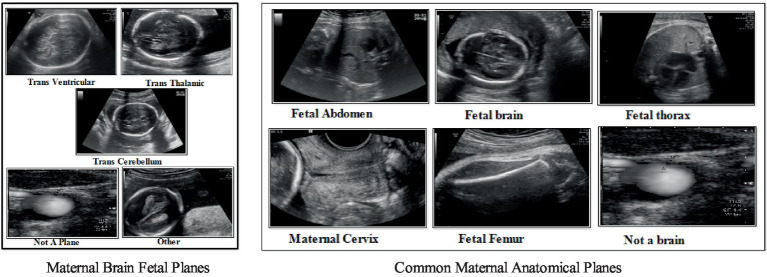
A few sample images of common maternal fetal and fetal brain ultrasound images.
